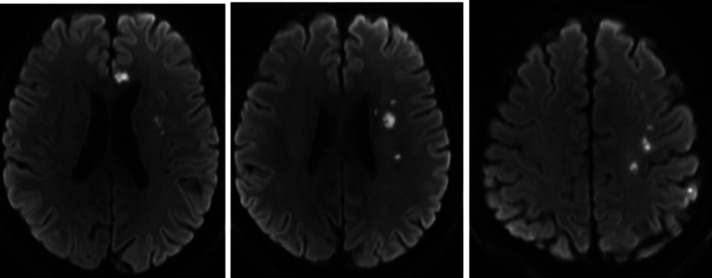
Admission brain magnetic resonance imaging showing acute cerebral infarction.
